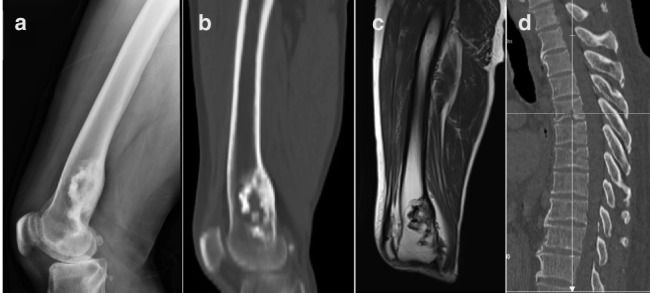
A 63‐year‐old male farmer presented with a history of gradually progressive right leg pain which he had experienced for 1 year. The pain initially only occurred during activity, but developed into persistent sharp pain. After 1 month of worsening pain, he was admitted to our hospital. On physical examination, the distal right femur was tender, with no warmth or erythema. Routine blood examination results were normal. (a) Radiography and (b) computed tomography showed expansive bone destruction with surrounding osteosclerosis in the distal right femur. (c) Magnetic resonance imaging showed that the edge of the lesion was “map‐like.” (d) Computed tomography showed old bone destruction of the thoracic vertebrae
